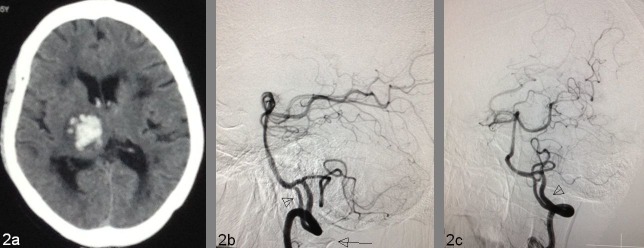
Intracranial vertebral artery fenestration in a 54-year-old man with a right thalamic bleed 2A: Plain CT scan shows a right thalamic bleed. 2B: VA fenestration involving both intracranial (arrowhead) and extracranial segments in lateral view on selective left VA angiography, the long arrow indicating the bony impression of the posterior arch of the C1 vertebra. 2C: Oblique view of VA fenestration (arrowhead). Length/width of the right limb is 20.7 mm/2.2 mm and of the left limb is 33.5 mm/3.1 mm. VA diameters distal to fenestration is 3.4 mm and proximal measurement is 3.9 mm.
